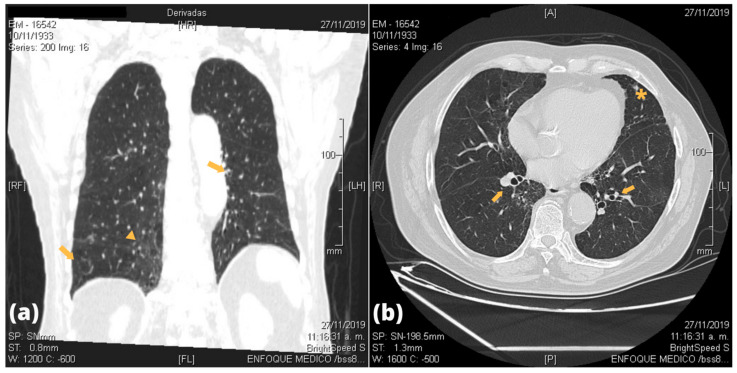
Images of an 86 years-old male patient with TSP/HAM and neurogenic bladder. (a) Coronal CT scan shows diffuse ground-glass pattern (arrowheads) in the right paramediastinal region, elevation of the left hemidiaphragm and presence of bronchiectasis (arrows). (b) Transverse CT scan shows diffuse ground-glass pattern, bronchiectasis (arrows), and presence of a nodular image (asterisk).
